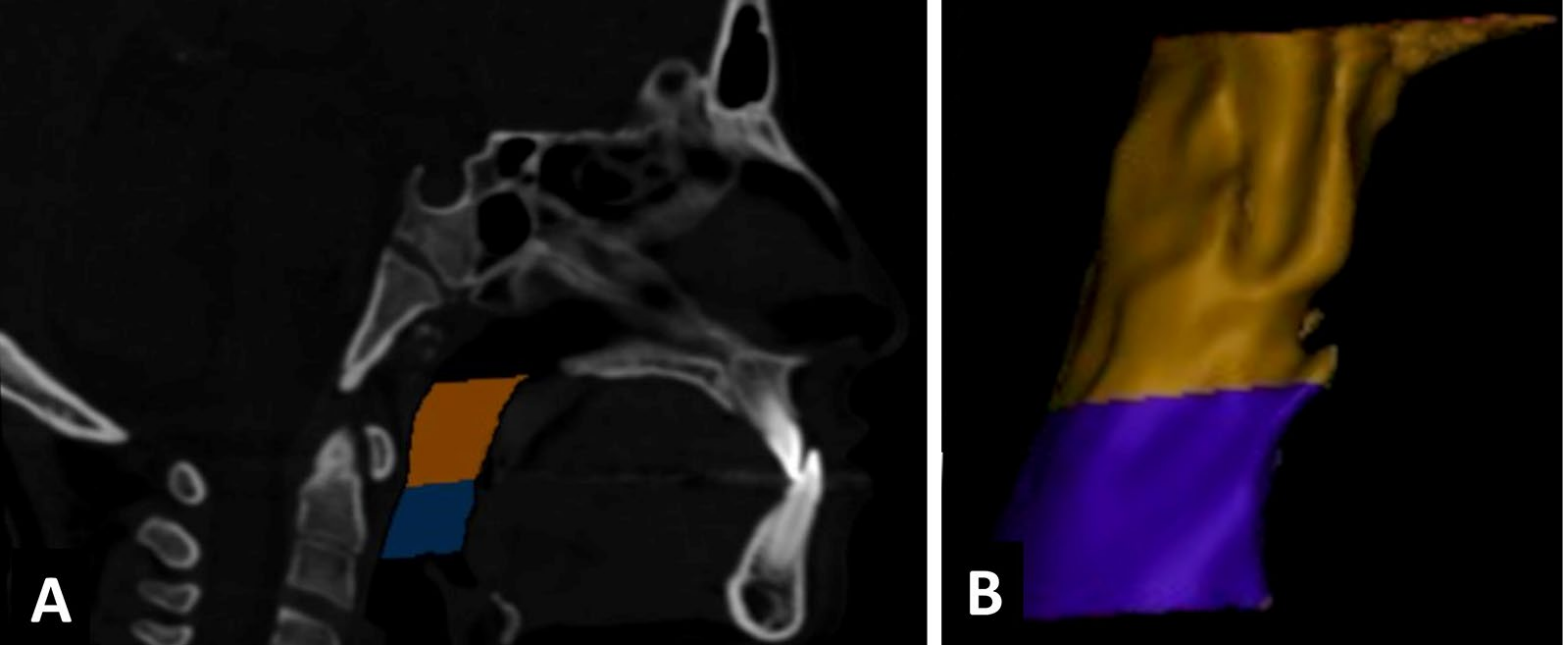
CT midsagittal image slice of the airway segmentation (a). Lateral view showing retropalatal and retroglossal 3D parts (b)
